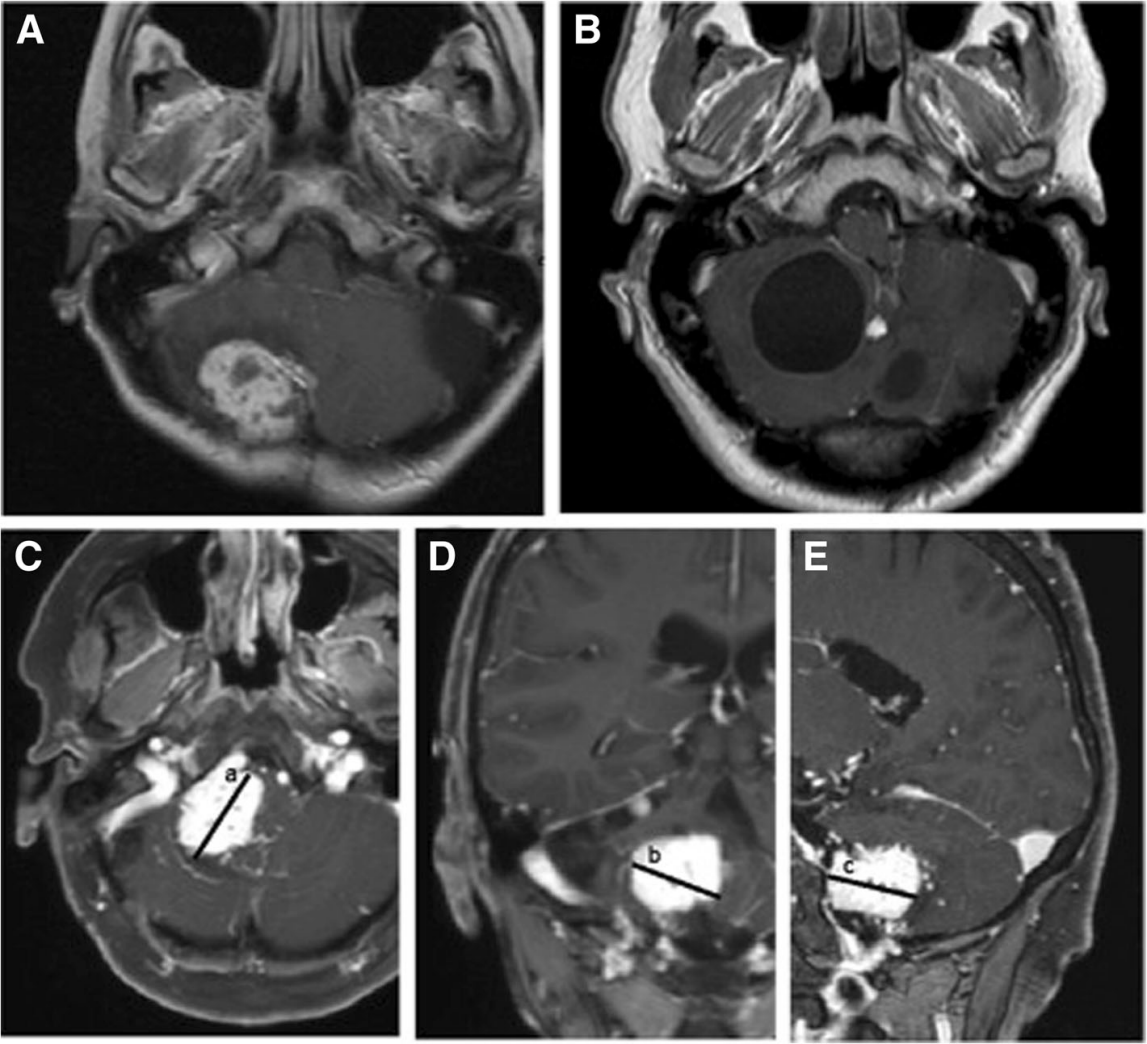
Examples of hemangioblastomas and measurement of solid tumor size in MRI; MRI scans with example of (a) solid tumor and (b) associated tumor cyst. Example of measurement of solid tumor size in contrast-enhanced MRI (c) axial, (d) coronar and (e) sagittal. Values a, b, c were multiplied and divided by 2 to receive tumor size volume in cm^3^
